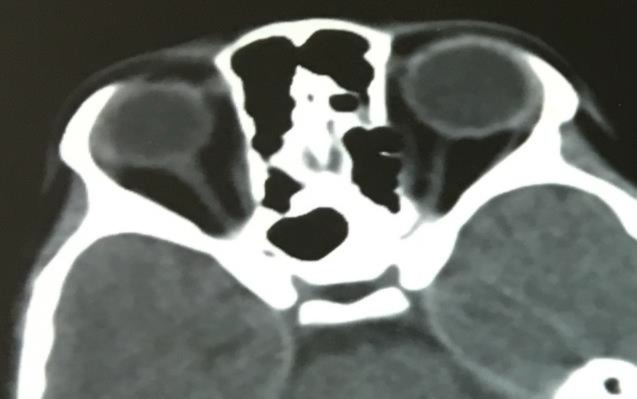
1-month postoperative CT without negative sequelae on both orbits and nasal cavities
